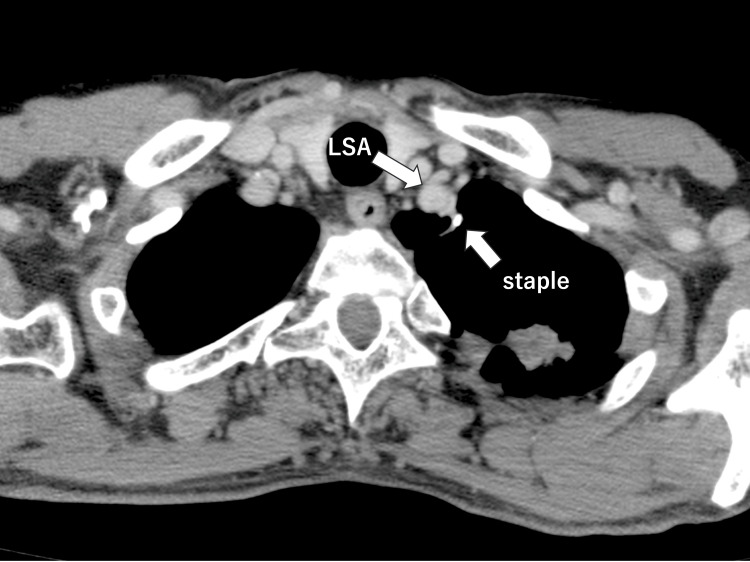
Review of the preoperative CT scan The left subclavian artery (LSA) ran very close to the stapler site of the previous bullectomy.
